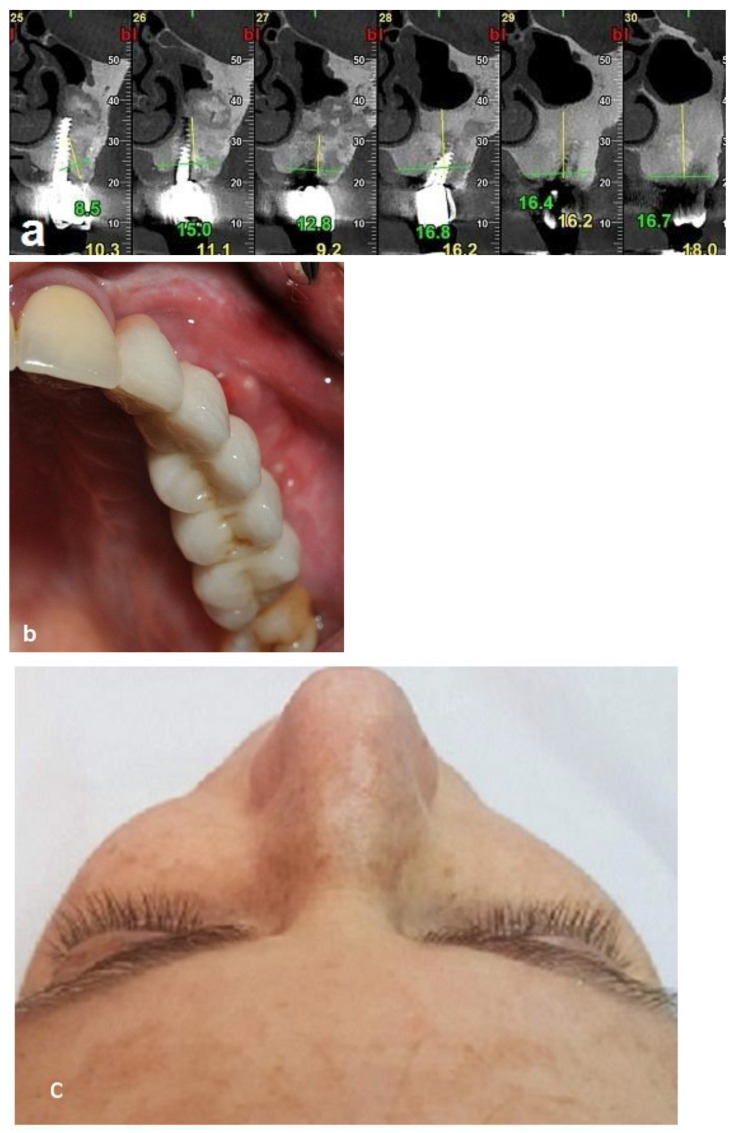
Clinical and radiographic images 7 years after the treatment show that there is no worsening of the FD lesion compared to the first picture that was obtained 7 years ago. (a) The CBCT view shows that the Bio-oss had been at least partially substituted by the FD lesion. (b) Intraoral view. (c) Extraoral views showing stable FD.
